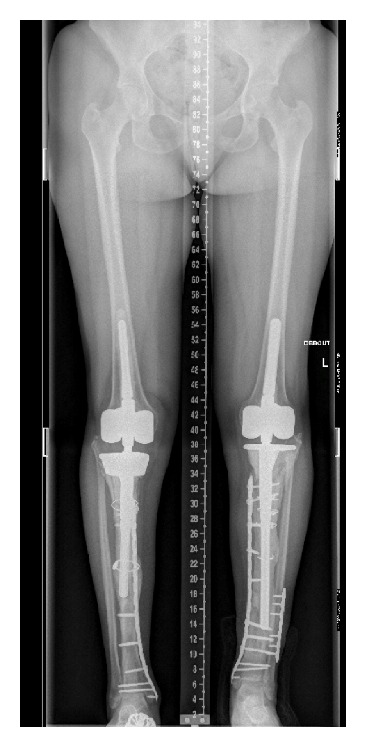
Follow-up X-rays, twelve months after osteosynthesis of the right tibial periprosthetic fracture.
